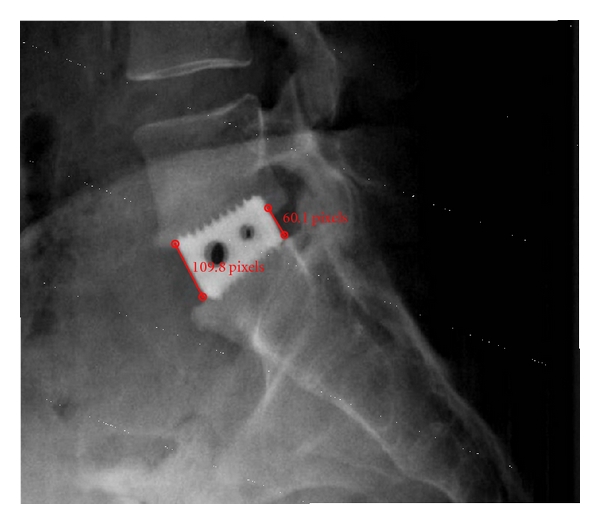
Measurement of scanned analog X-ray.
